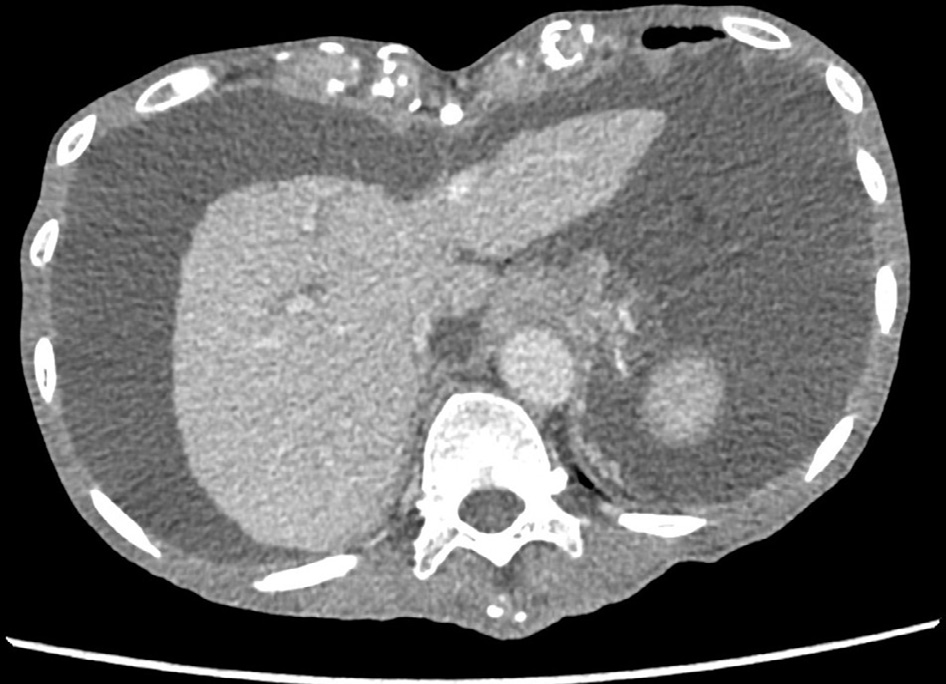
Contrast-enhanced axial CT abdomen shows a small and cirrhotic liver with gross ascites.
